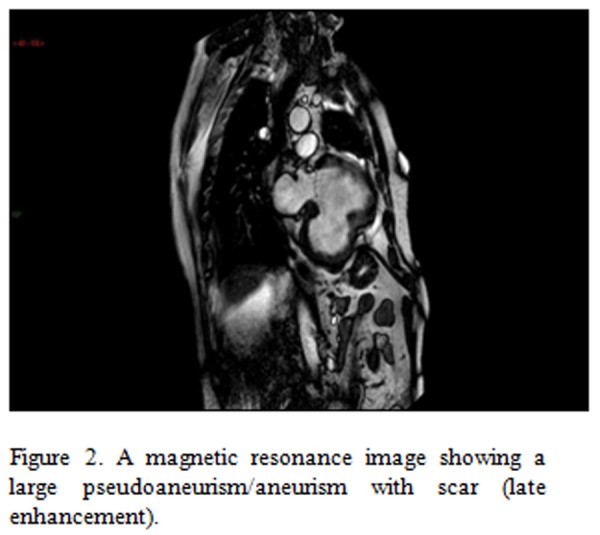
A magnetic resonance image showing a large pseudoaneurism/aneurism with scar (late enhancement).
